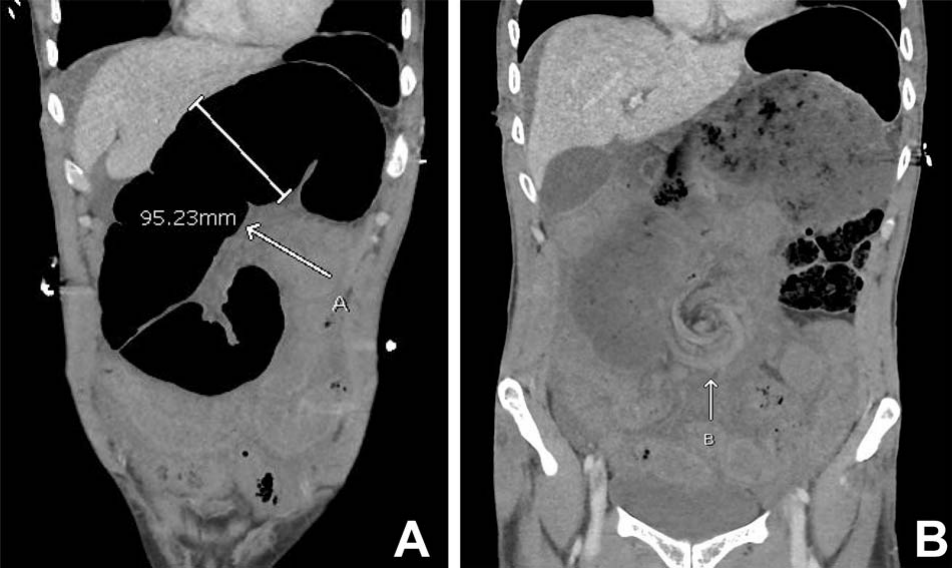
Initial CT abdomen pelvis with contrast in the portal venous phase showed (A) dilated small bowel and the (B) classical whirlpool sign.
